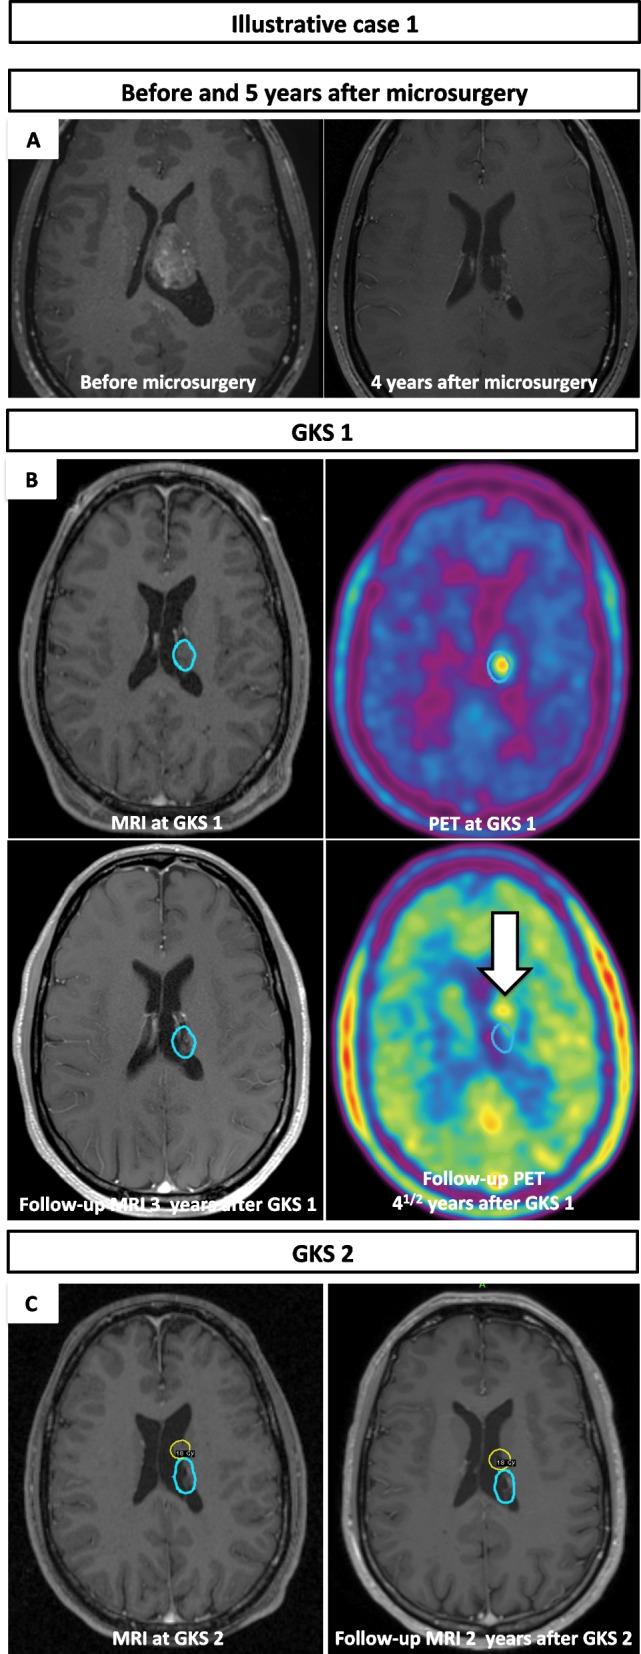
A Preoperative and 4 years after microsurgery MRI; B MRI and F-18 FET at the time of first GKS (upper image), and 3 years later (lower image); C MRI at the time of second GKS (left image) and 2 years later (right), with major shrinkage. Arrow points toward the recurrence which will be further treated by a second GKS
